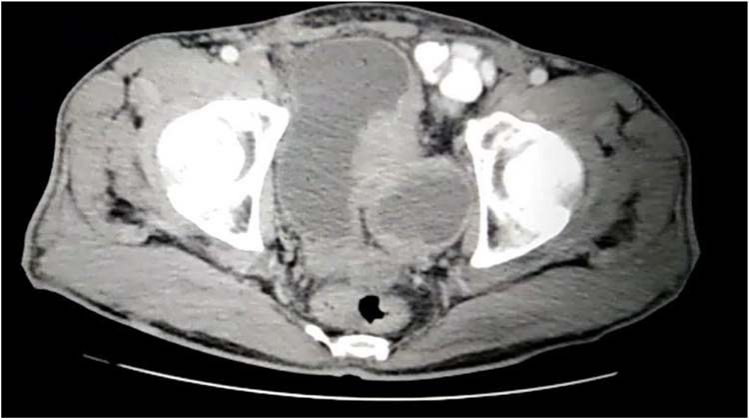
CT showing a large bladder diverticulum on the posterior wall with a mass within it.
